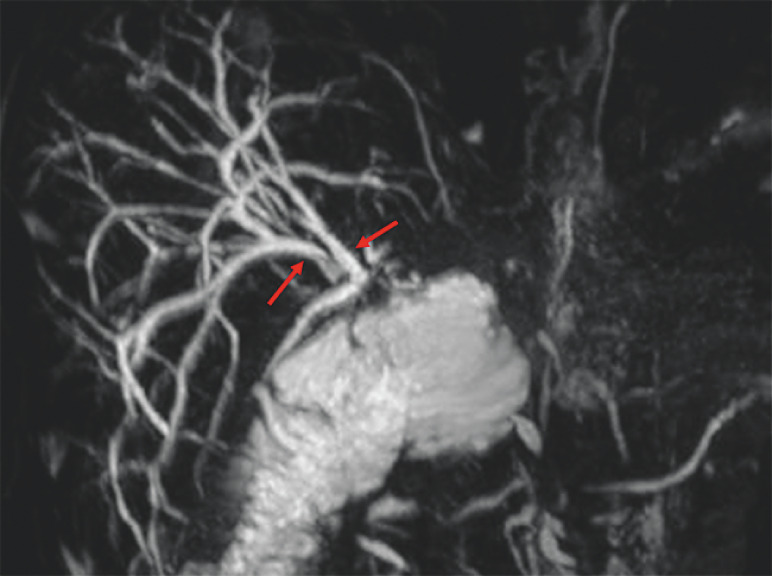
Magnetic resonance imaging revealing slight biliary dilation (red arrow) in the intrahepatic bile duct.
